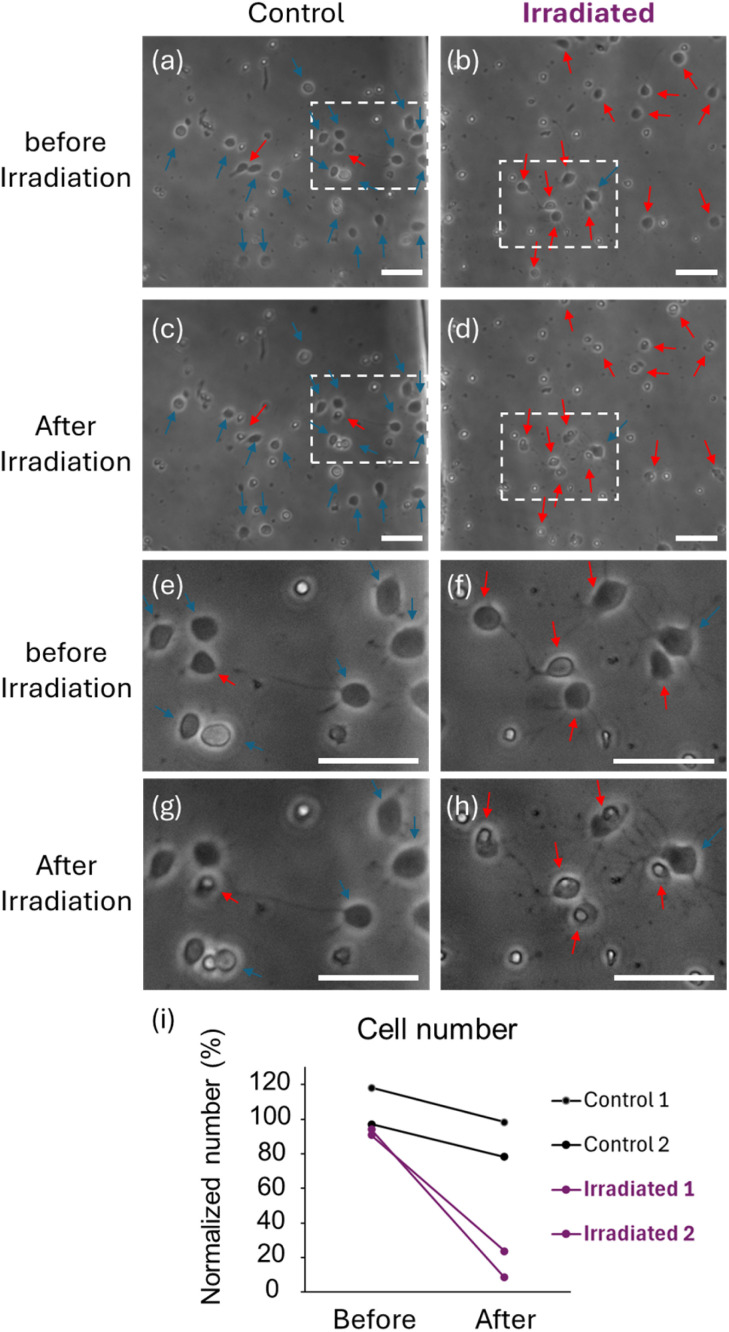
Induction of cell death by focal irradiation of X-rays to one of the two chambers of the CPMS. (a and b) Cells in the chamber before X-ray irradiation. (c) Cells in the chamber 6 h after focal irradiation of X-rays to the chamber shielded by lead plates. The position in the chamber of the CPMS used for image acquisition in (c) was the same as that in (a). (d) Cells in the chamber 6 h after focal irradiation of X-rays without shielding with the lead plate. The position in the chamber for image acquisition in (d) was the same as that in (b). (e, f, g and h) Enlarged images of hippocampal cells in the squares of the images in (a, b, c and d), respectively. In the area shielded by the lead plate, most of the cells did not show pyknotic nuclei (blue arrows, white small spots) 6 h after X-ray irradiation. In the unshielded chamber, many cells became pyknotic (red arrows) 6 h after irradiation, indicating preferential induction of cell death in the irradiated chamber. The scale bars indicate 50 µm. (i) The number of hippocampal cells normalized to the average cell number in the fields before irradiation. The cell number in the chamber without shielding was greatly reduced after irradiation (irradiated 1 and 2) compared with that in the chamber with shielding (control 1 and 2). Each circle indicates the normalized number of live cells.
